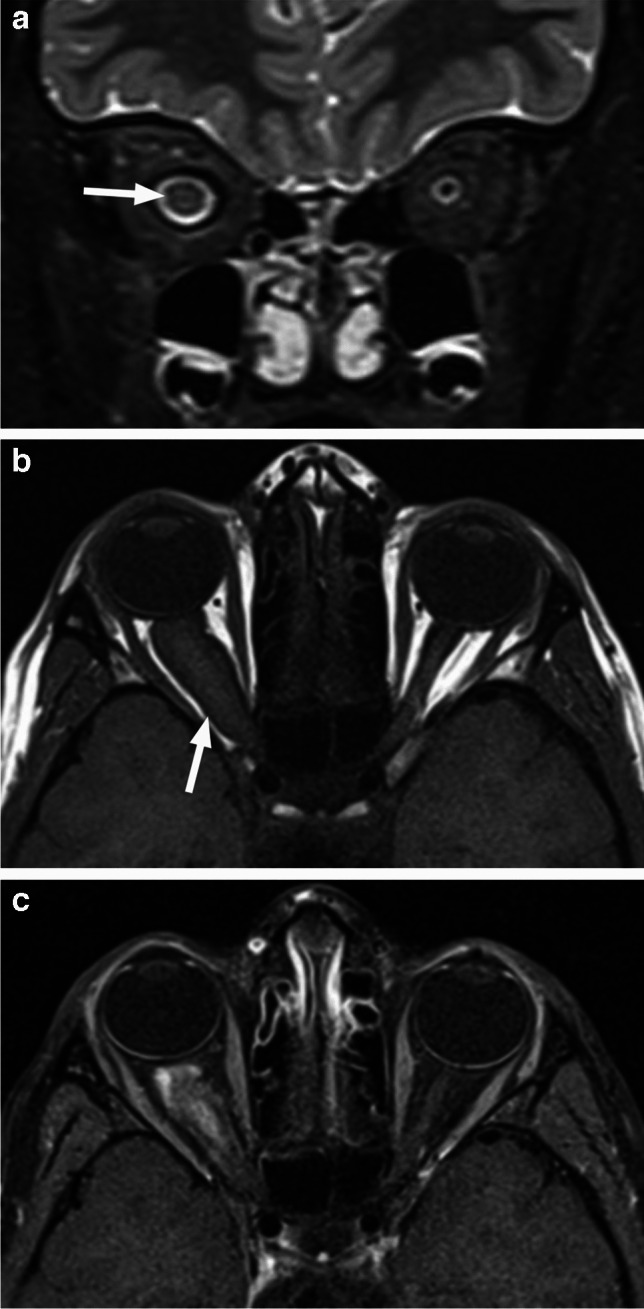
Optic nerve glioma. Coronal STIR image through the orbits (a) reveals a very prominent thickening and slightly increased signal intensity of the right optic nerve (arrow). Axial T1-weighted image (b) exhibits fusiform enlargement of the entire intraorbital nerve (arrow), which demonstrates patchy enhancement on the corresponding postcontrast T1-weighted image with fat saturation (c)
